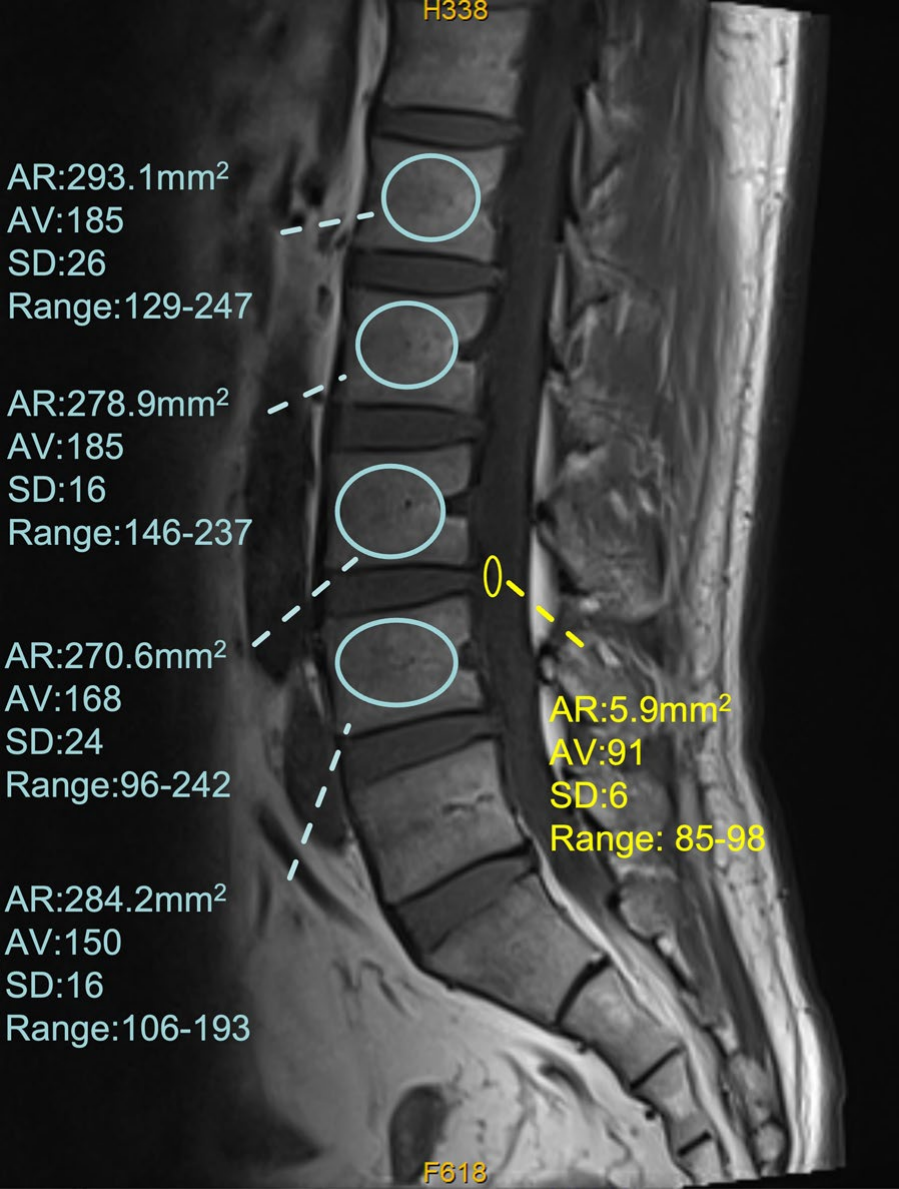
Non−contrast-enhanced T1-weighted MRI of the lumbar spine detailing the regions of interest (circles) used to calculate VBQ score; AR= area ratio; AV = average signal intensity of the region of interest; SD= standard deviation of signal intensity of the region-of-interest (VBQ score in shown example=2.291)
